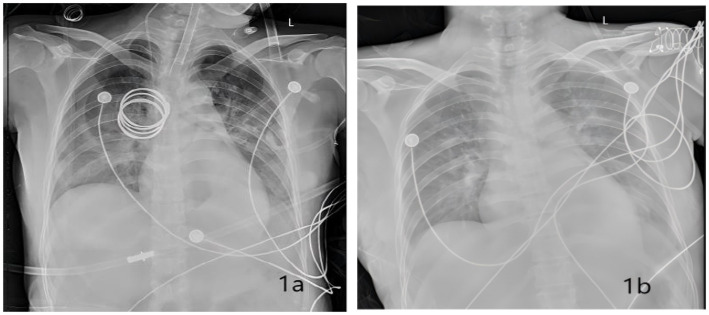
Bedside chest X-ray film findings of the patient. (a) Bilateral pulmonary exudation demonstrated on postpartum day 2. (b) Bilateral pulmonary exudation still demonstrated on postpartum day 5.
